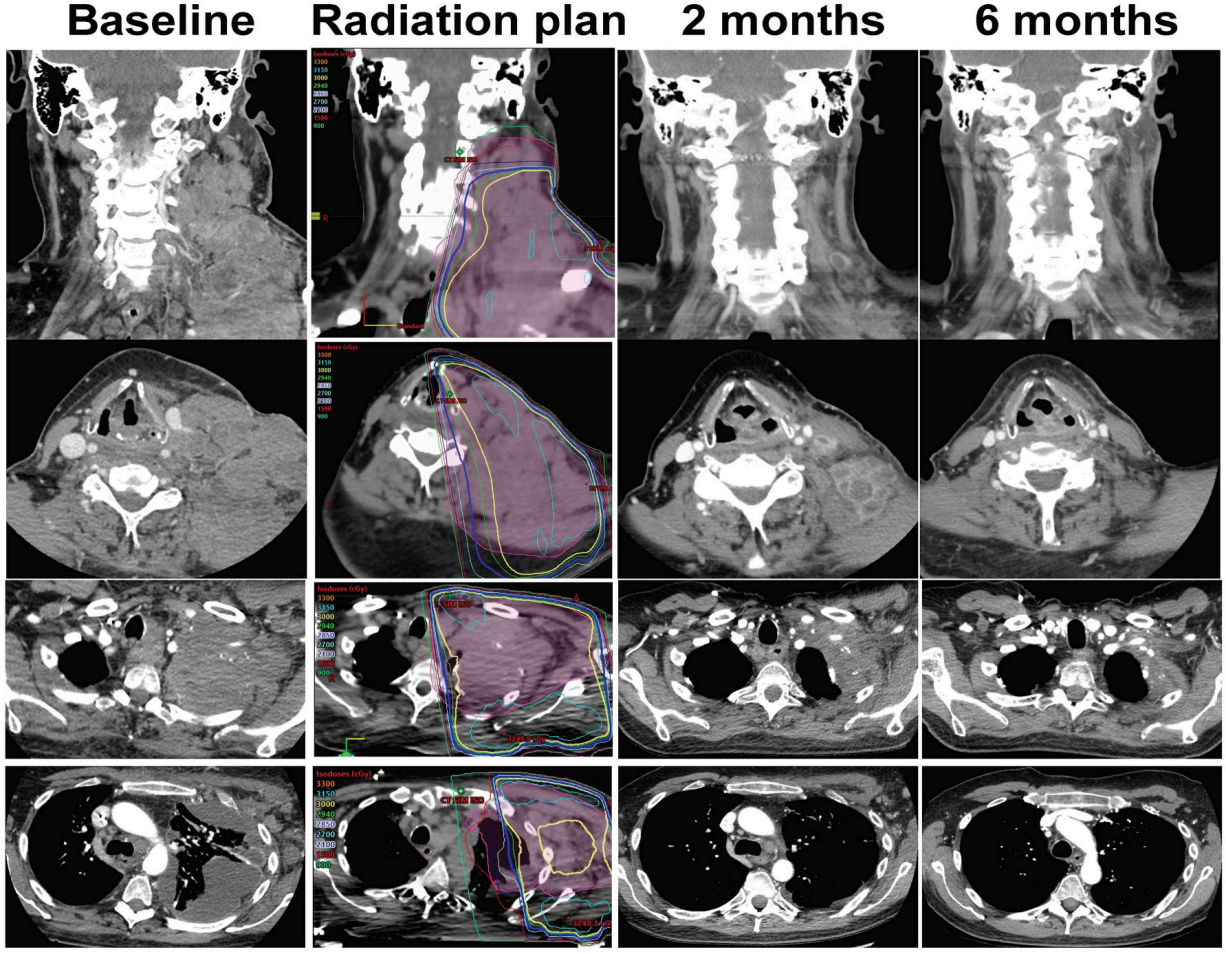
CT imaging of patient treated with RT plus nivolumab. Excellent partial response at 2 months and near complete response at 6 months of the lesions in the left neck, supraclavicular region, lung apex, pleural, and axillar disease. Response was maintained for 13 months.
